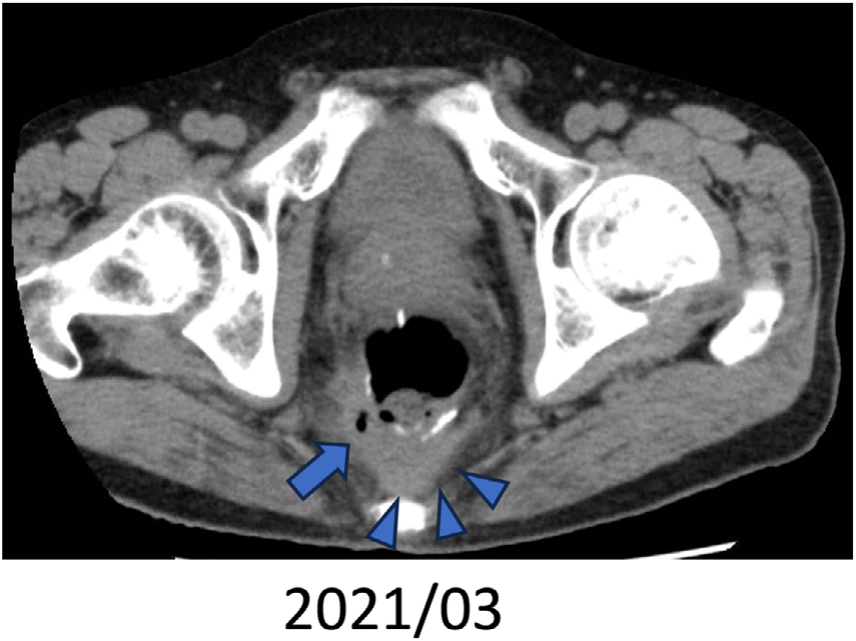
Image of computed tomography. Fluid retention on the dorsal side of the anastomosis.
